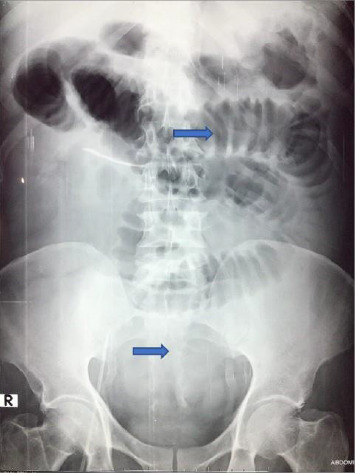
Case 2: Radiograph of abdomen (anteroposterior view) showing dilated multiple small bowel loops in central abdomen (arrow) with collapsed large bowel without free gas in peritoneal cavity, suggestive of small intestinal obstruction.
